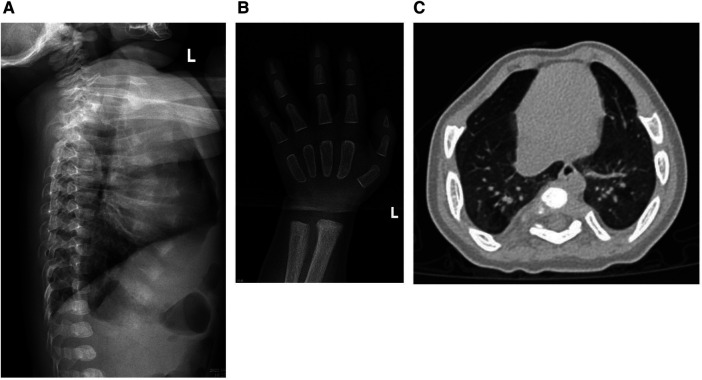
(A) The x-ray shows the spine have kyphosis at the junction of the thoracolumbar segment. Thoracic and lumbar physiological curvature developmental abnormalities. (B) The cartilage development of wrist joint was abnormal. (C) The chest was deformed and the interstitial changes of the lungs were obvious.
